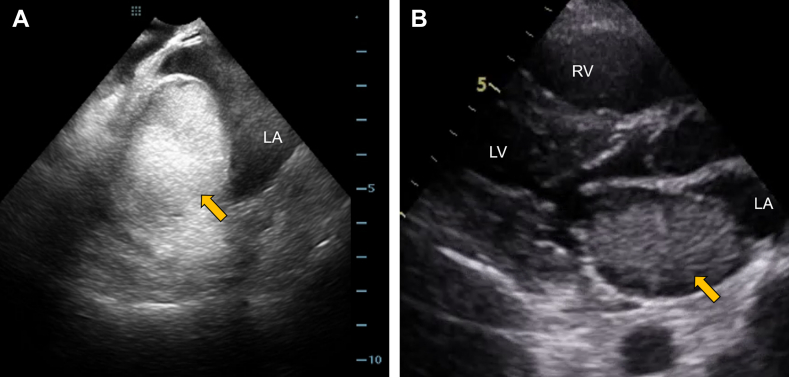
Echocardiographic Images of the Left Atrial Intramural Hematoma (A) Intracardiac echocardiography showed a high echoic structure in the left atrium (arrow). (B) TTE showed a mass occupying most of the left atrium cavity (arrow). LA = left atrium; LAIH = left atrial intramural hematoma; LV = left ventricle; RV = right ventricle; TTE = transthoracic echocardiography.
